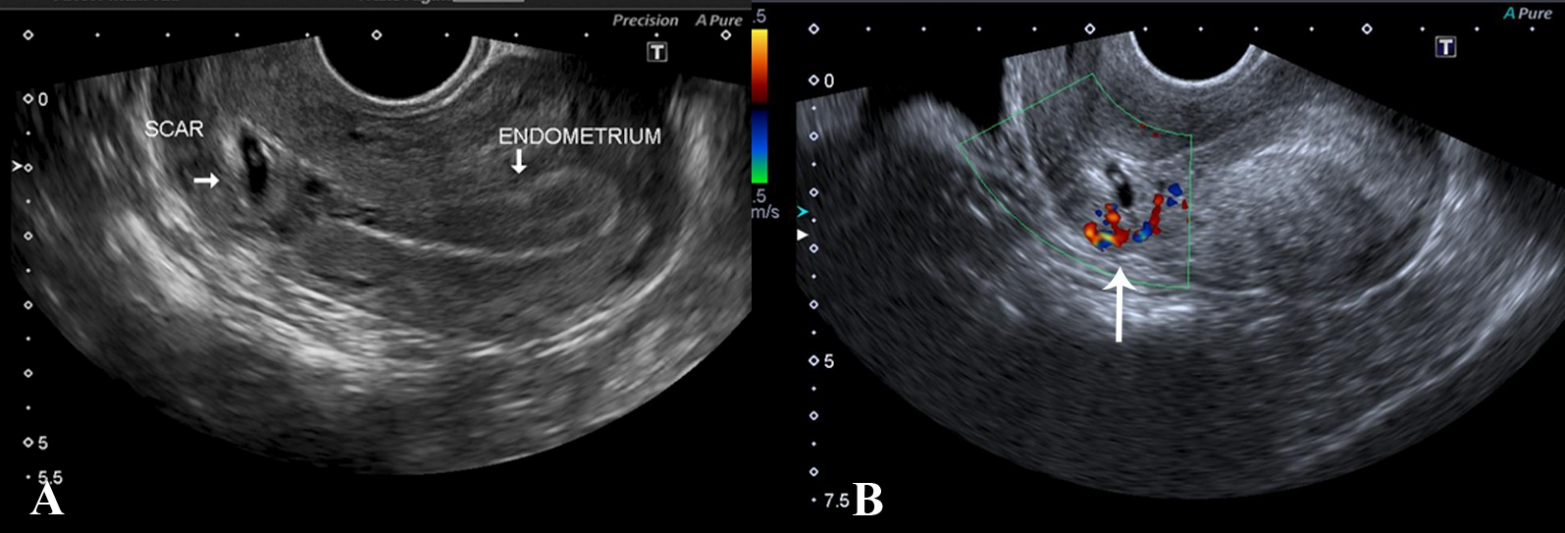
(A) TVS showing retroverted uterus with empty endometrial cavity and low-lying gestational sac containing fetal pole, close to the scar site. (B) Increased vascularity was noted around the gestational sac on Doppler (arrow). TVS: Transvaginal scan
